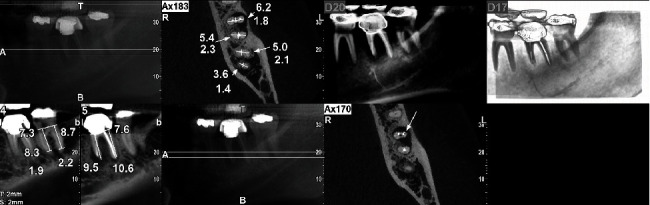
Cone-beam computed tomography (CBCT) showed large periradicular radiolucency around the mesial root in tooth #18, with sizable bone loss/resorption and the furcation involvement.
